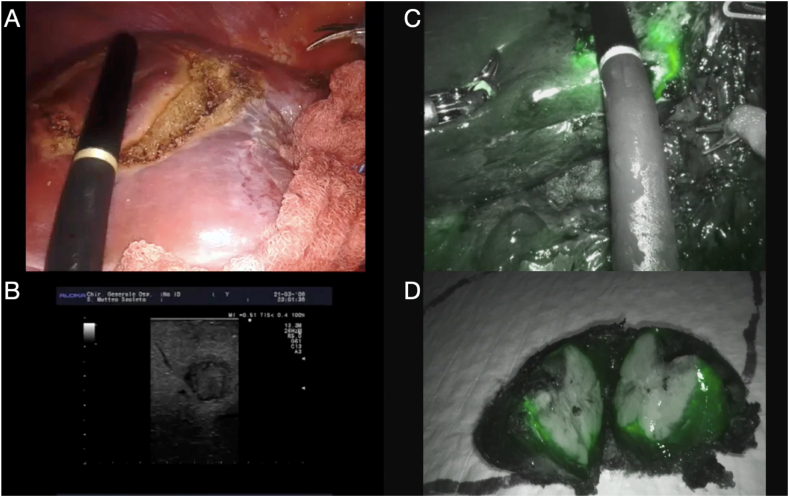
A-B. Intraoperative UltraSound-guided wedge resection to perform parenchymal sparing and achieve R0 margins; C-D. Accumulation of Indocyanine Green (ICG) around liver metastases after preoperative injection to evaluate margin status and recognize subcapsular metastases.
